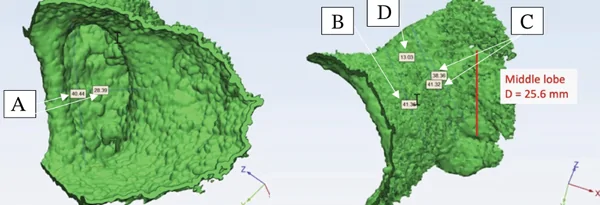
3D CT reconstruction of left atrial appendage in long axis and short axis views (A) Ostium 40.44 mm (long axis) and 28.39 mm (short axis), (B) ostium 41.36 mm with landing zone (C) 38.36–41.32 mm, and (D) length between ostium and landing zone of 13.03 mm (posterior to ostium).
